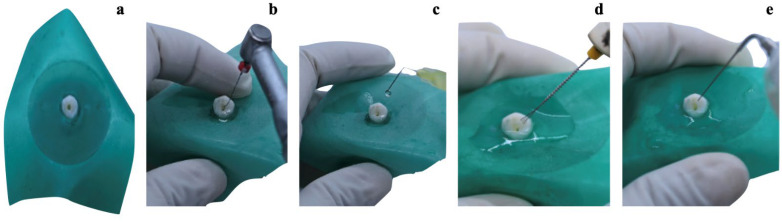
Irrigation agitation/activation techniques: (a) a gel model setup along with rubber dam, (b) the final agitation using an XP Endo Finisher, (c) the final agitation using a side-vented needle, (d) the final agitation using a Gentle File Finisher Brush, and (e) the final agitation using Passive Ultrasonic Activation.
